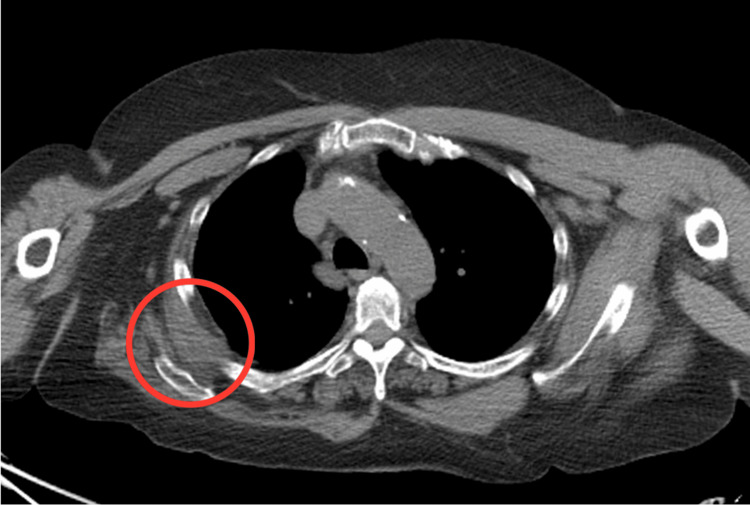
Chest CT (plain) revealing a soft tissue mass (red circle) along the lower angle of the scapula and subjacent ribs
